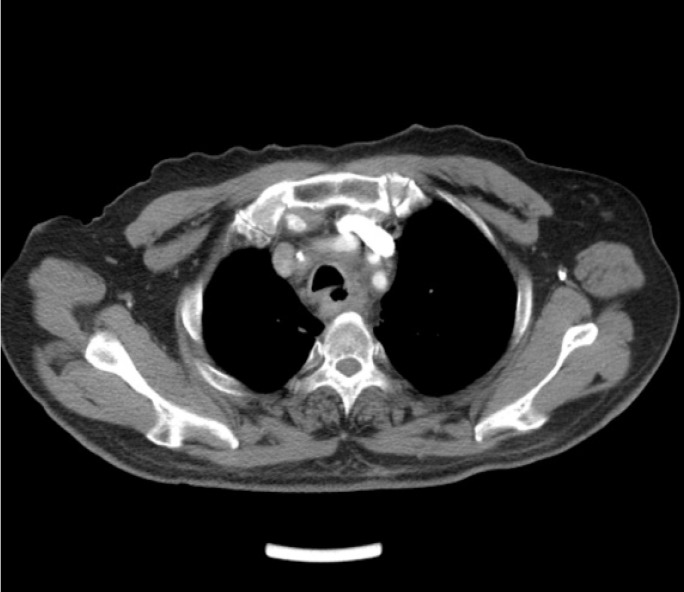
Axial contrast-enhanced computed tomographic (CT) scan of the chest shows esophagorespiratory fistula.
